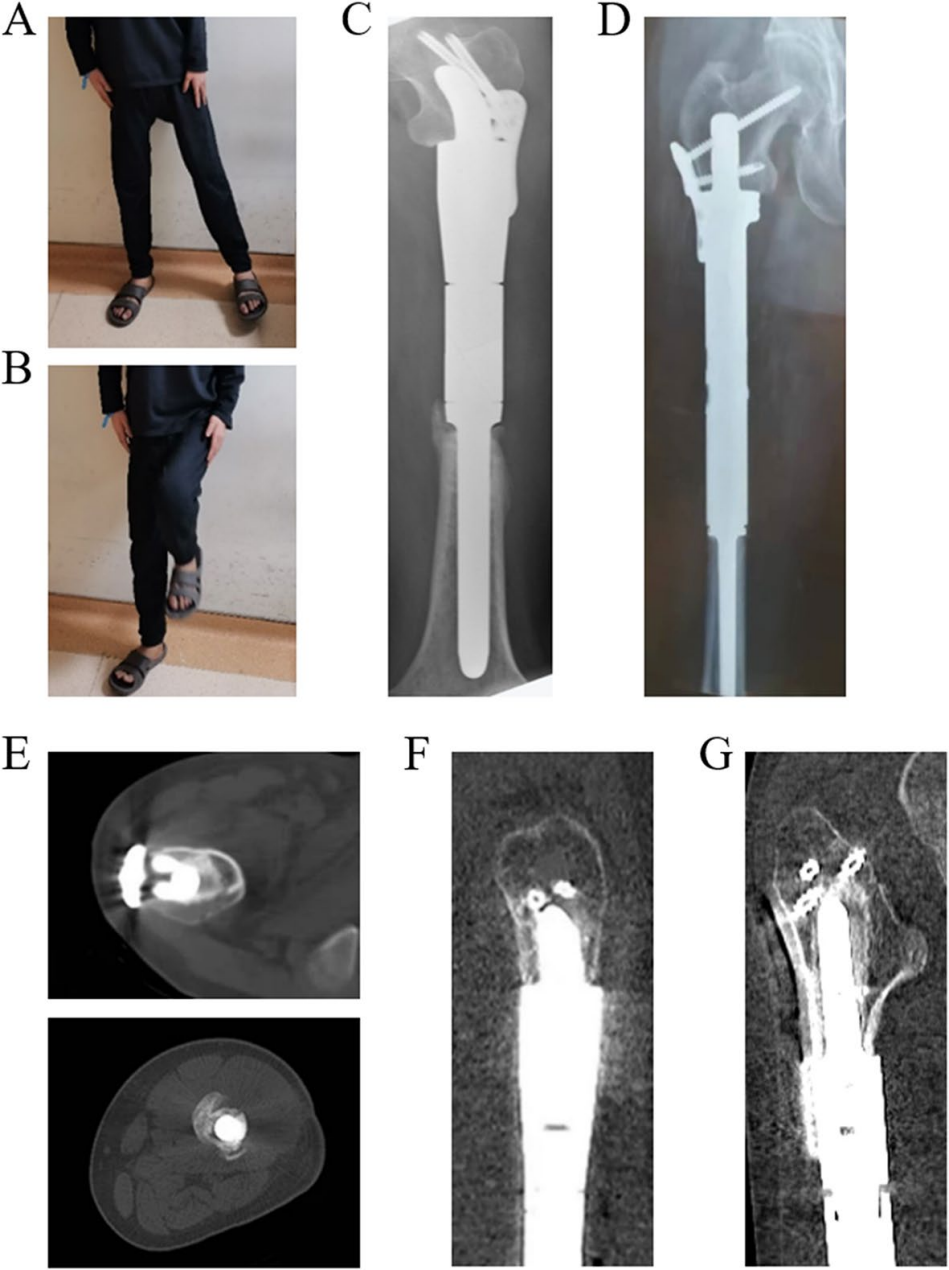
The postoperative functional and radiographic outcomes in the follow-up. A and B The normal hip range of motion of abduction A and flexion B in Patient 9 at five months after surgery. C X-ray of the left femur at 99 days after surgery of Patient 1 showing reliable biological fixation. D X-ray of the right femur at 85 days after surgery of Patient 3 showing reliable biological fixation. E Bone ingrowth on the bone-implant interface of the 3D-printed stem and screw (Patient 6) or osteotomy plane (Patient 1) at six months after surgery on axial CT image. F Bone ingrowth on the bone-implant interface of the 3D-printed curved stem and screws at six months of Patient 1 after surgery on reconstructed sagittal CT image. G Bone ingrowth on the bone-implant interface of the 3D-printed anchorage-slot stem and screws at six months of Patient 6 after surgery on reconstructed coronal CT image
